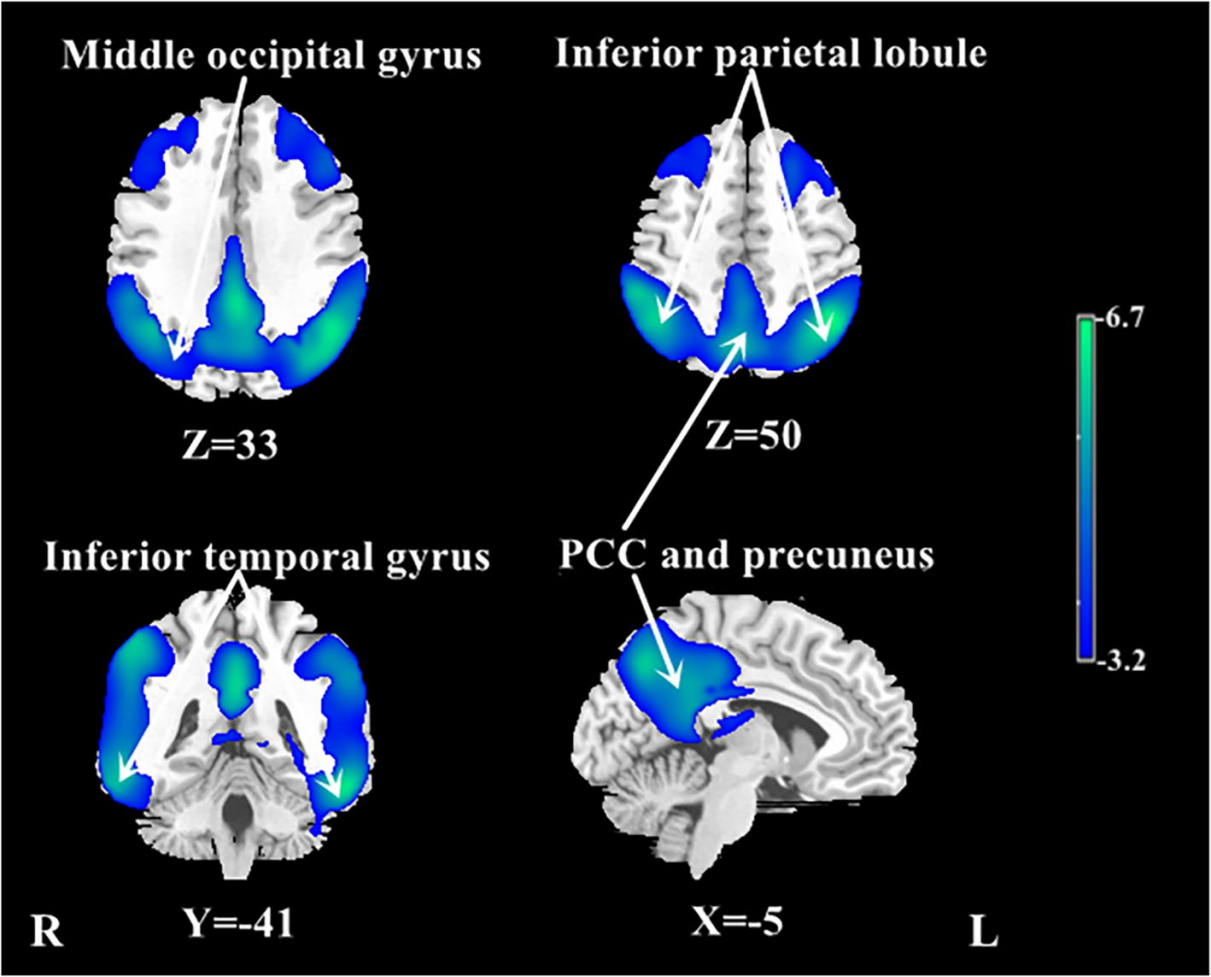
Regional CBF changes in patients with AD from univariate analysis without adjusting for the global value in the identification cohort. Cool color indicates regions with decreased CBF in AD patients compared with NCs. A threshold of 3.23 (*P* < 0.001, uncorrected) was used to overlay SPM maps onto a standard MRI brain template. PCC posterior cingulate cortex
